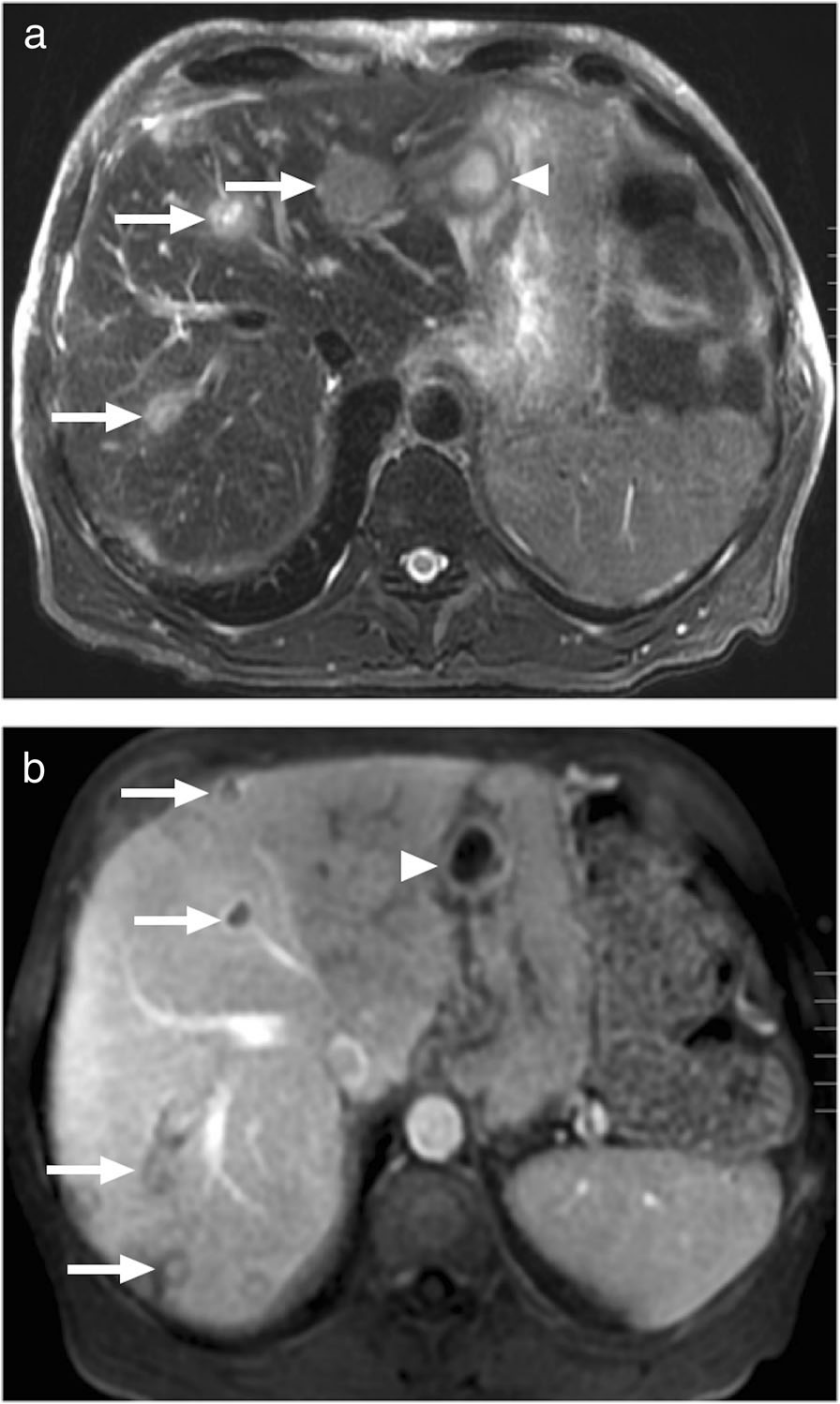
A 66-year-old male with poorly controlled diabetes presented with abdominal pain, fever and weight loss. a Axial plane T2W MR image demonstrates hyperintense, predominantly cystic lesions (arrows). Also note is made of necrotic lymph node in the gastrohepatic ligament (arrowhead). b Axial plane postcontrast T1W MR image demonstrates intense peripheral contrast enhancement (arrows). Percutaneous biopsy confirmed actinomyces infection.
